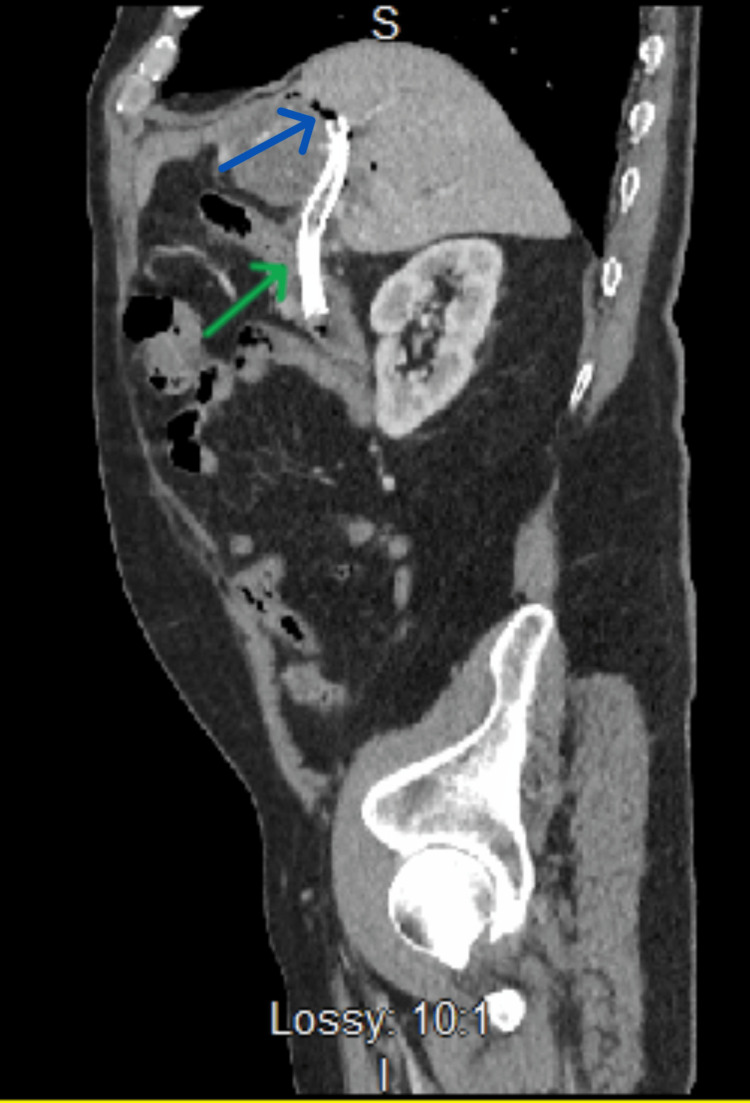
Sagittal CT image of the abdomen. There is mild pneumobilia (blue arrow) and the stent is in place (green arrow).
